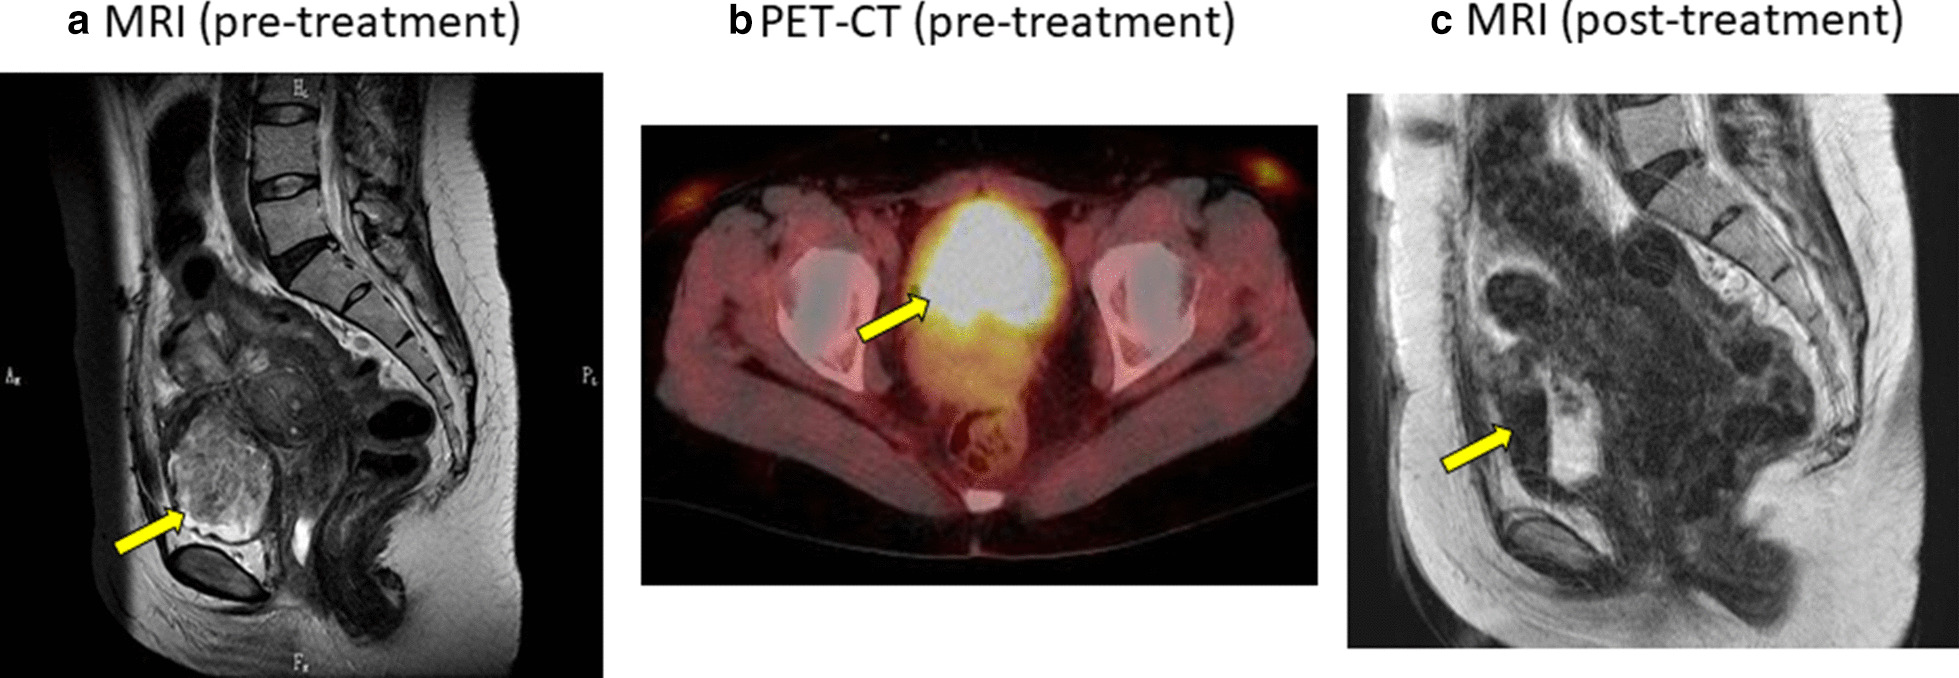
Bladder mass by a pre-treatment MRI (T2-weighted), b pre-treatment PET-CT, c post-treatment MRI (T2-weighted)
